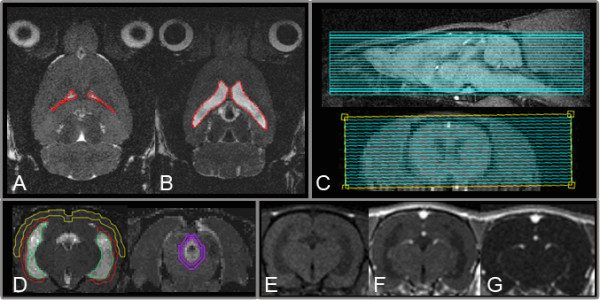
MRI imaging. A T2-weighted fast spin-echo sequence was used for quantification of lateral ventricular volume (A: control, B: 2W kaolin). Twenty-two contiguous T2-weighted axial slices were acquired in interleaved order, as shown in C. For quantification of water diffusion, four different regions of interest (cortex (yellow), periventricular (red), periaqueductal (purple) and CSF (green)) were drawn, using the b = 0 images, and apparent diffusion coefficient values were calculated for each ROI (D). In addition, T_1_-weighted pre (E) and post (F) contrast images were acquired. Blood brain barrier integrity was determined by subtraction of pre-contrast images from post-contrast images (G).
